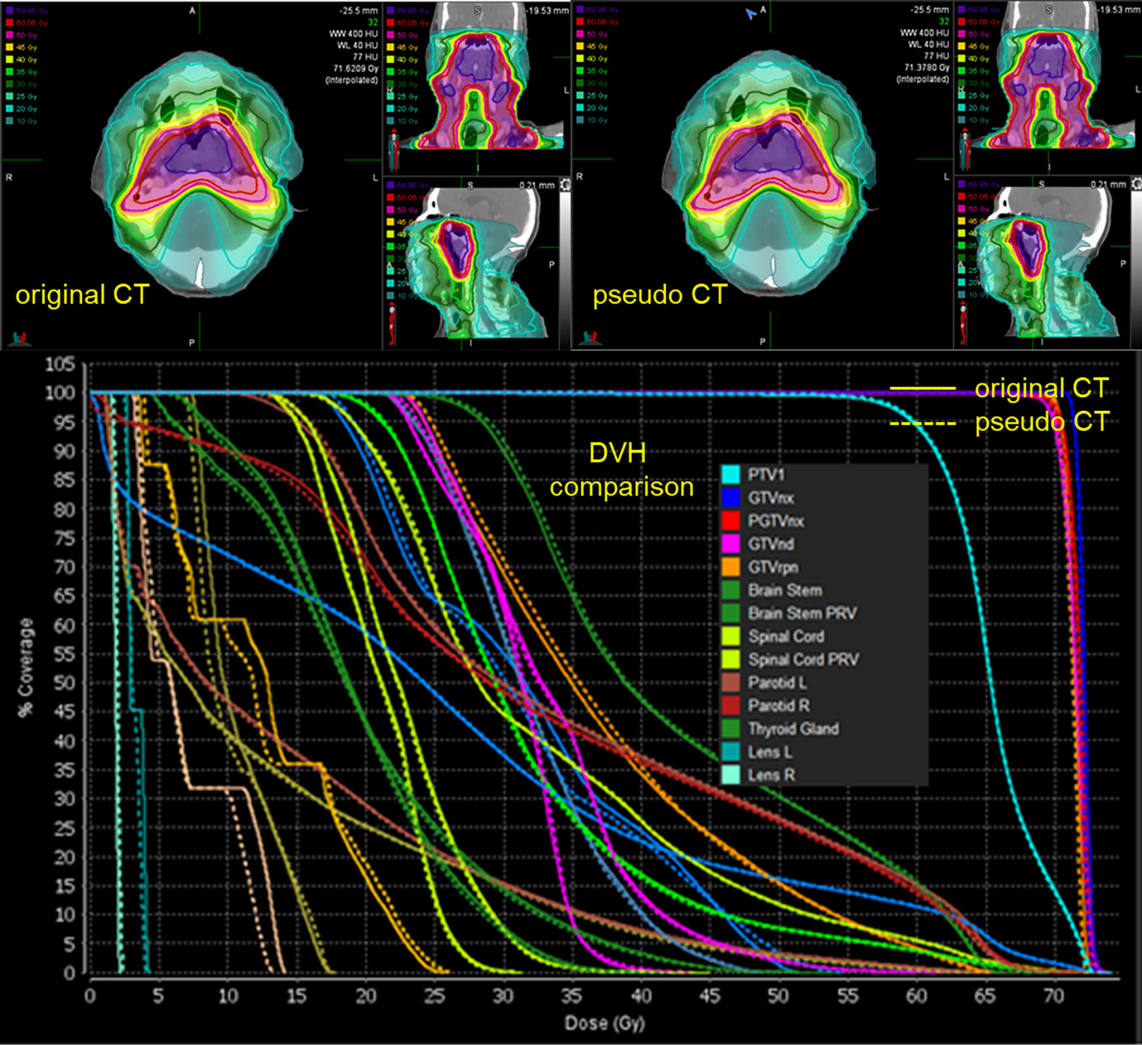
Spatial dose distributions of the original CT (left-up panel) and pCT (down-left panel) with identical beam assignment and their DVH comparison. The solid lines in the DVH correspond to the original CT, and the dotted lines correspond to pCT.
